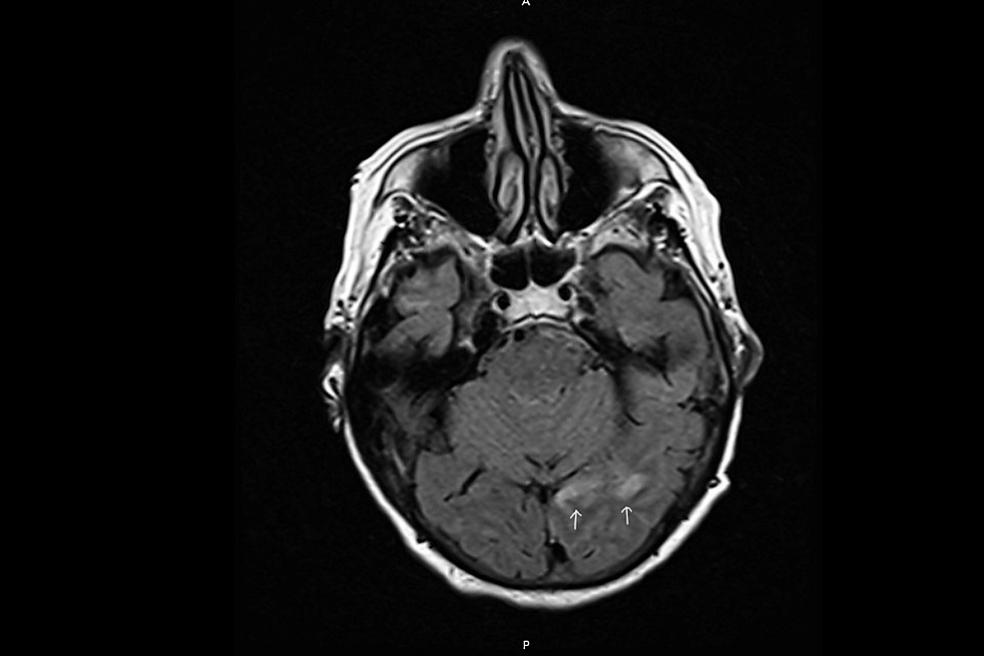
Magnetic resonance imaging of the brain without contrast. Magnetic resonance imaging (MRI) of the brain without contrast is demonstrating an area of acute/subacute stroke (white arrows) involving the medial margins of the left occipital and left posterior temporal lobe. No associated hemorrhagic transformation is demonstrated in the area of the stroke.
